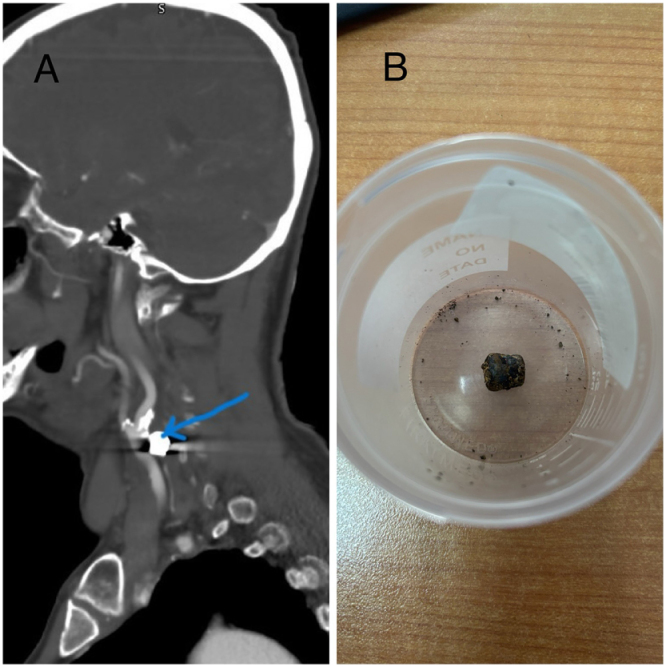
(A) Preoperative CTA showing critical right ICA stenosis (90%) with a metallic artifact near the carotid bifurcation (patient image). (B) İntraoperative photograph of the extracted bullet fragment after removal from beneath the ICA–ECA bifurcation (patient image).
